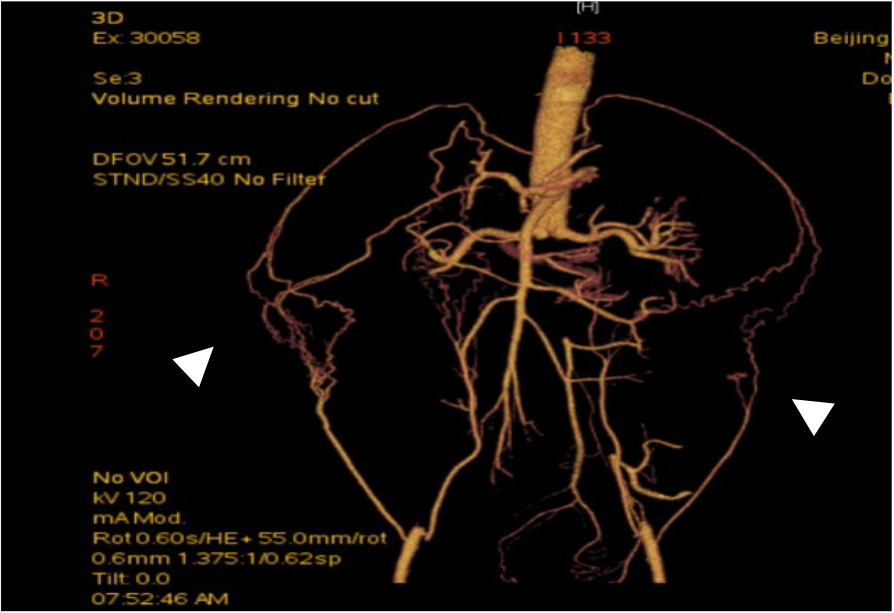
Computed tomography angiogram of the entire aorta showed diffuse calcified occlusions affecting infra-renal abdominal aorta to bilateral common iliac arteries and establishments of collateral vessels (indicated by white arrow heads)
